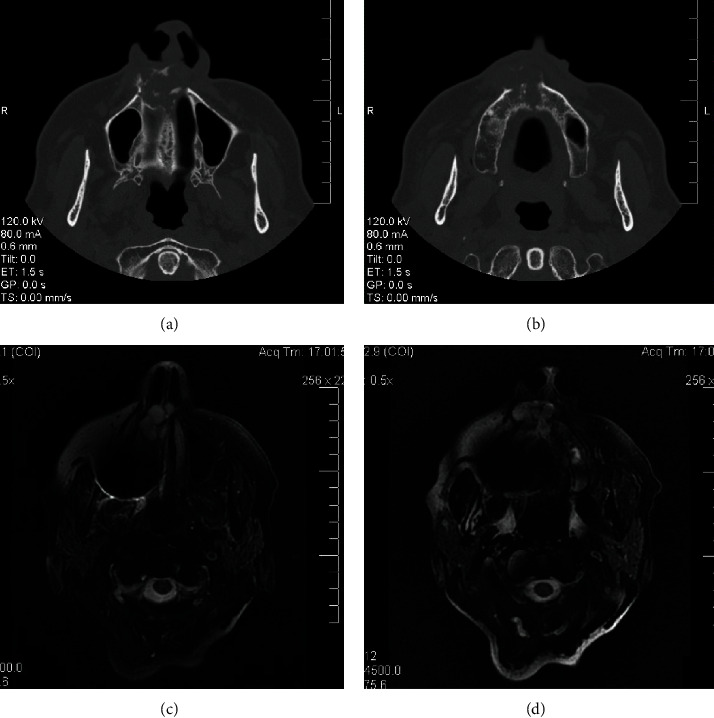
(a, b) Preoperative CT scan. (c, d) Preoperative MRI.
